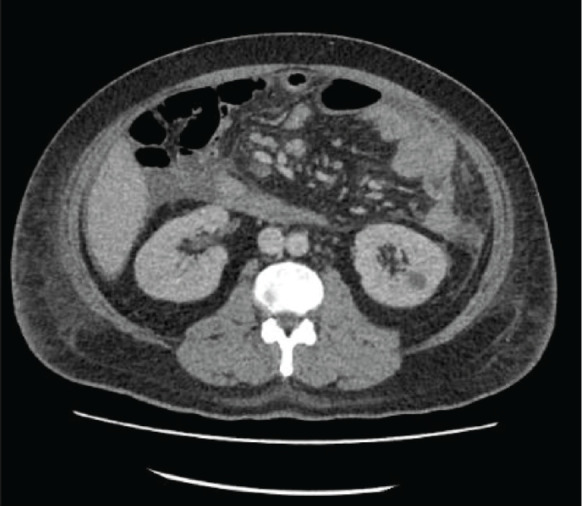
CT scan revealing a 5.6 cm neoplastic mass in the rectum and bilateral Bosniak 1 renal cysts.
